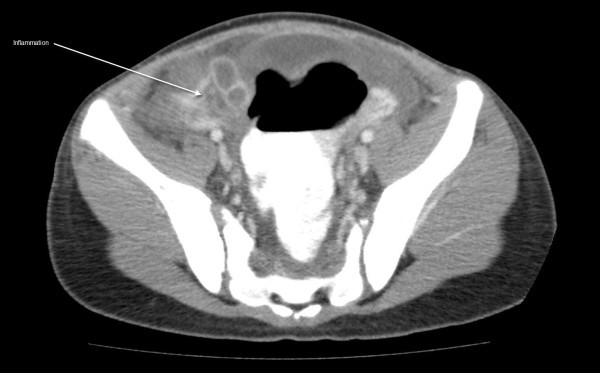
CT scan of the abdomen and pelvis with intravenous and oral contrast. Inflammation is demonstrated.
